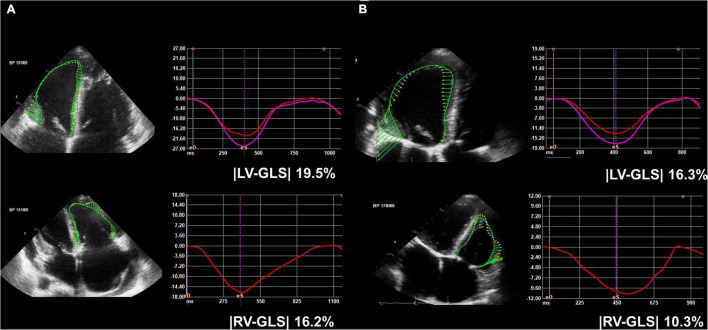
Representative figure of (A) pre-op and (B) post-op LV-GLS and RV-GLS measurements. LV-GLS was calculated from three standard apical images, and RV-GLS was calculated from RV focused apical echocardiographic images. LV, left ventricle; RV, right ventricle; GLS, global longitudinal strain; |LV-GLS|, absolute value of LV-GLS; |RV-GLS|, absolute value of RV-GLS.
